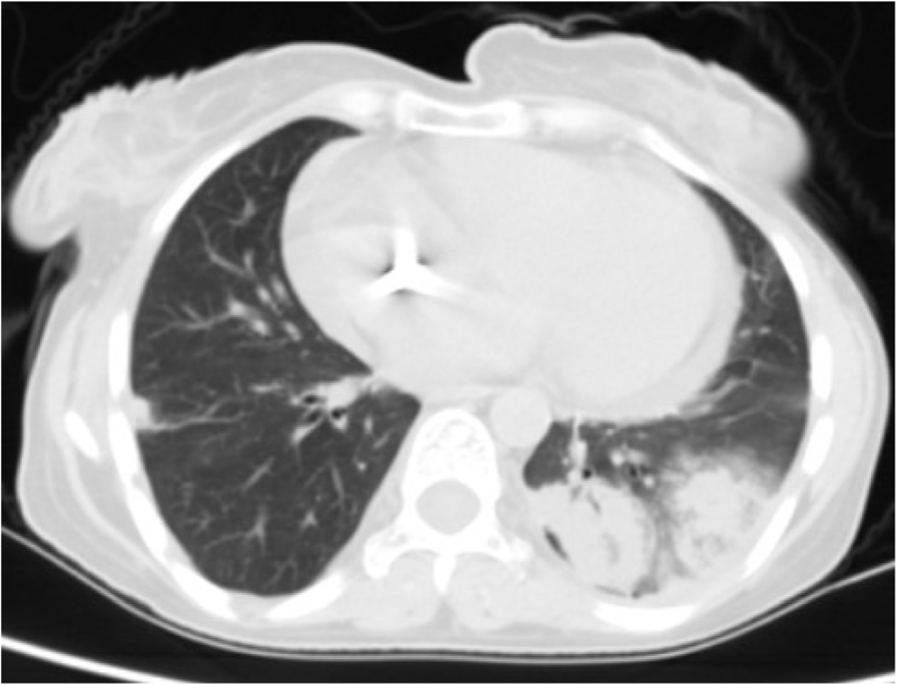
Angioinvasive aspergillosis represented by halo: thin section CT shows nodular area of consolidation with surrounding ground-glass opacities (halo sign) in left lower lobe
